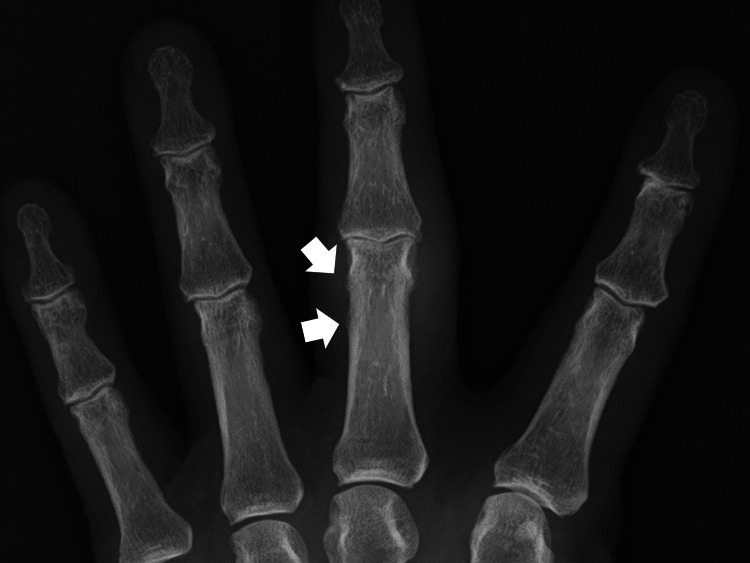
X-ray of the left hand showing osteophytes at the site of tendon attachment around the proximal interphalangeal joint (white arrows).
